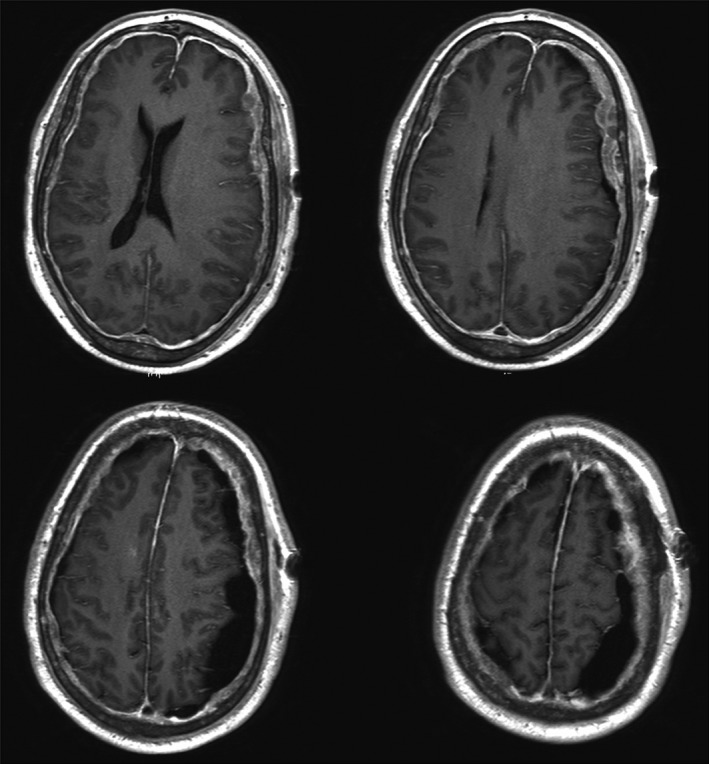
MRI of the brain with contrast reveals abnormal enhancement and thickening of the dura with resultant mass effect and midline shift.
